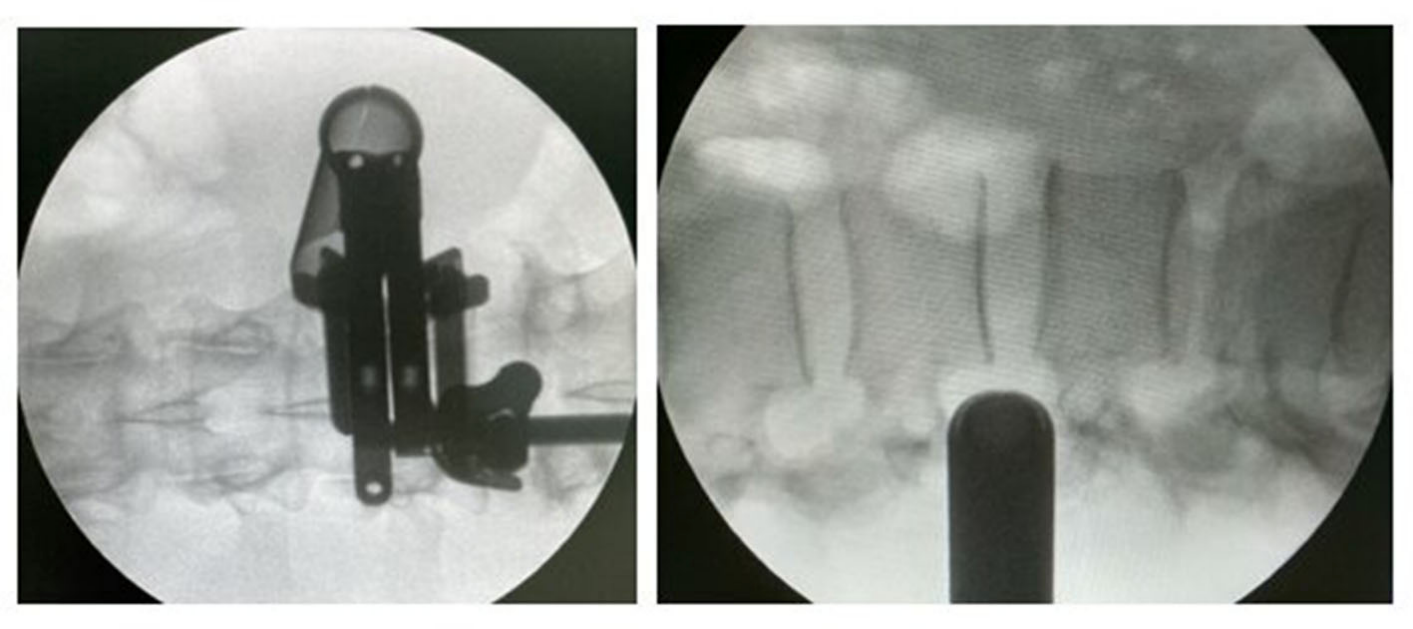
Direct and lateral X-ray control during the surgery.
